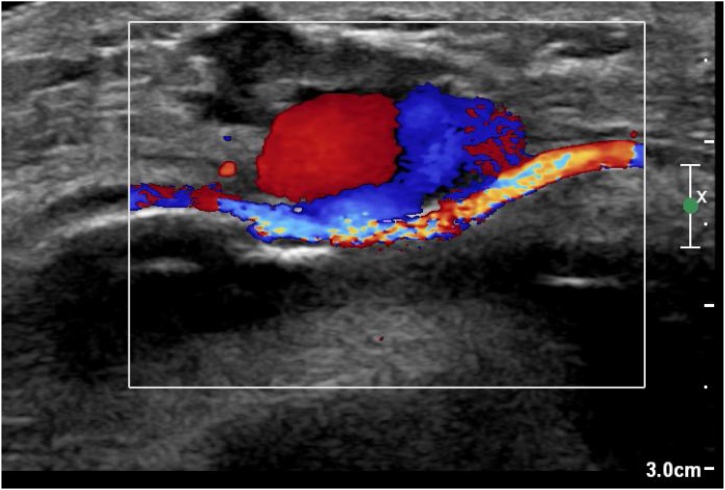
Ultrasonogrpahy showing the pulsatile mass with aliasing flow inside and the continuity with the artery with a narrow neck.
